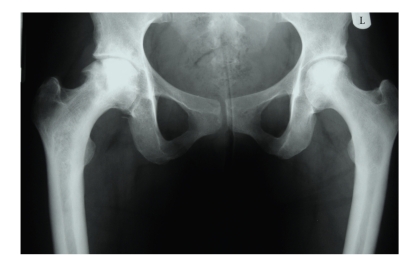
Plain radiograph of the pelvis in another patient with sickle cell anaemia more advanced avascular necrosis with sclerosis and subchondral collapse of the right femoral head. Less severe changes are seen on the left.
